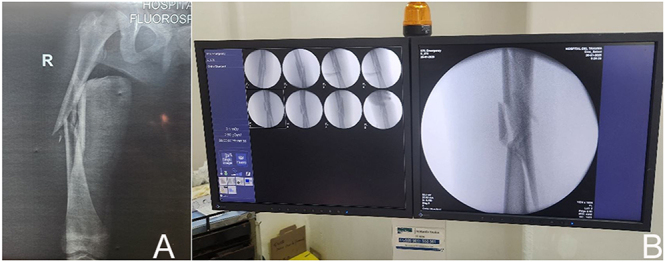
Preoperative radiograph (A) and fluoroscopic image following limb fracture (B).
